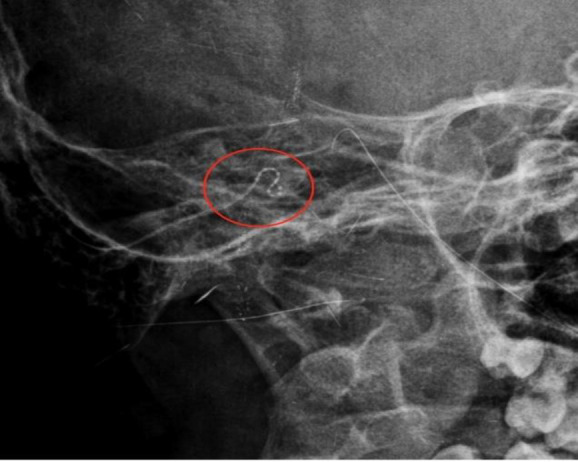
X-ray of misplacement of the electrode array in the internal acoustic canal
